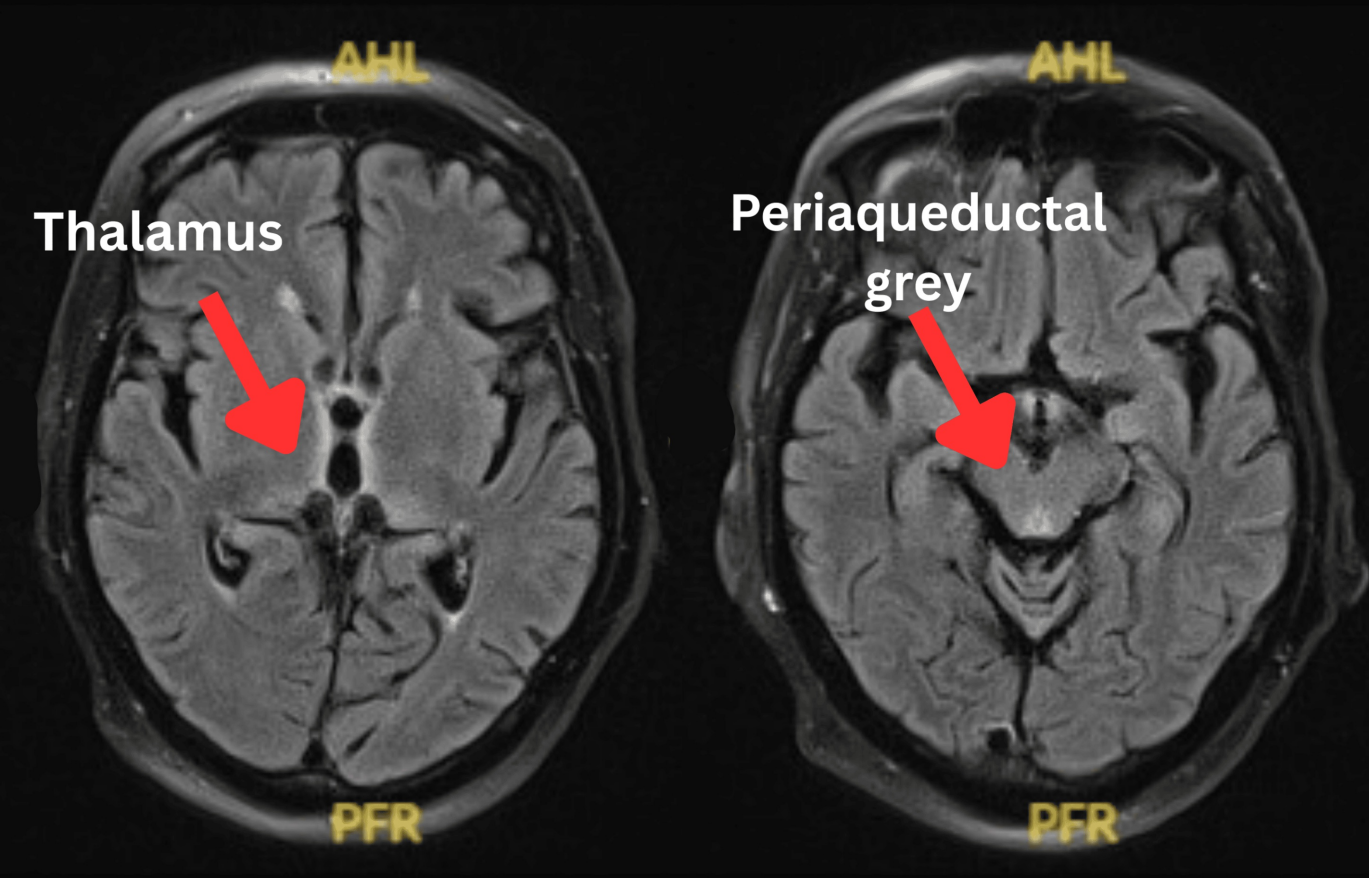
Follow-up MRI brain (axial FLAIR) five days after initiation of intravenous thiamine demonstrating marked regression of signal abnormalities. MRI: magnetic resonance imaging; FLAIR: fluid-attenuated inversion recovery; AHL: anterior horn of the left lateral ventricle; PFR: posterior frontal region.
